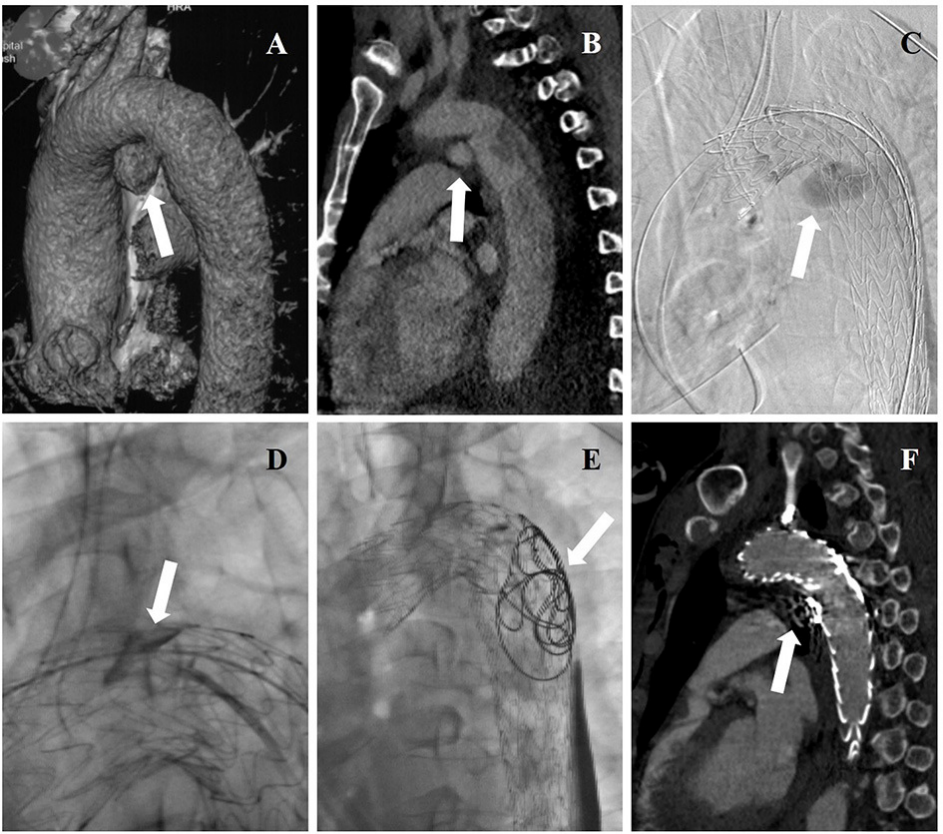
(A) Three-dimensional reconstructions of pre-operative CTA showing concomitant aortic arch pseudoaneurysm (arrow) of traumatic aortic dissection. (B) Pre-operative CTA showing the entry tear (arrow) of aortic dissection. (C) Intraoperative DSA showing deployment of aortic stent graft and CGs, and angiography through a pre-road catheter to FL revealed intraoperative EL-I (arrow). (D) Onyx glues were injected into gutters (arrow) through a microcatheter. (E) Coils and Onyx glues were deployed into FL (arrow) through a pre-road catheter. (F) The aortic dissection and pseudoaneurysm were entirely excluded while endoleak disappeared (arrow) in post-operative CTA.
